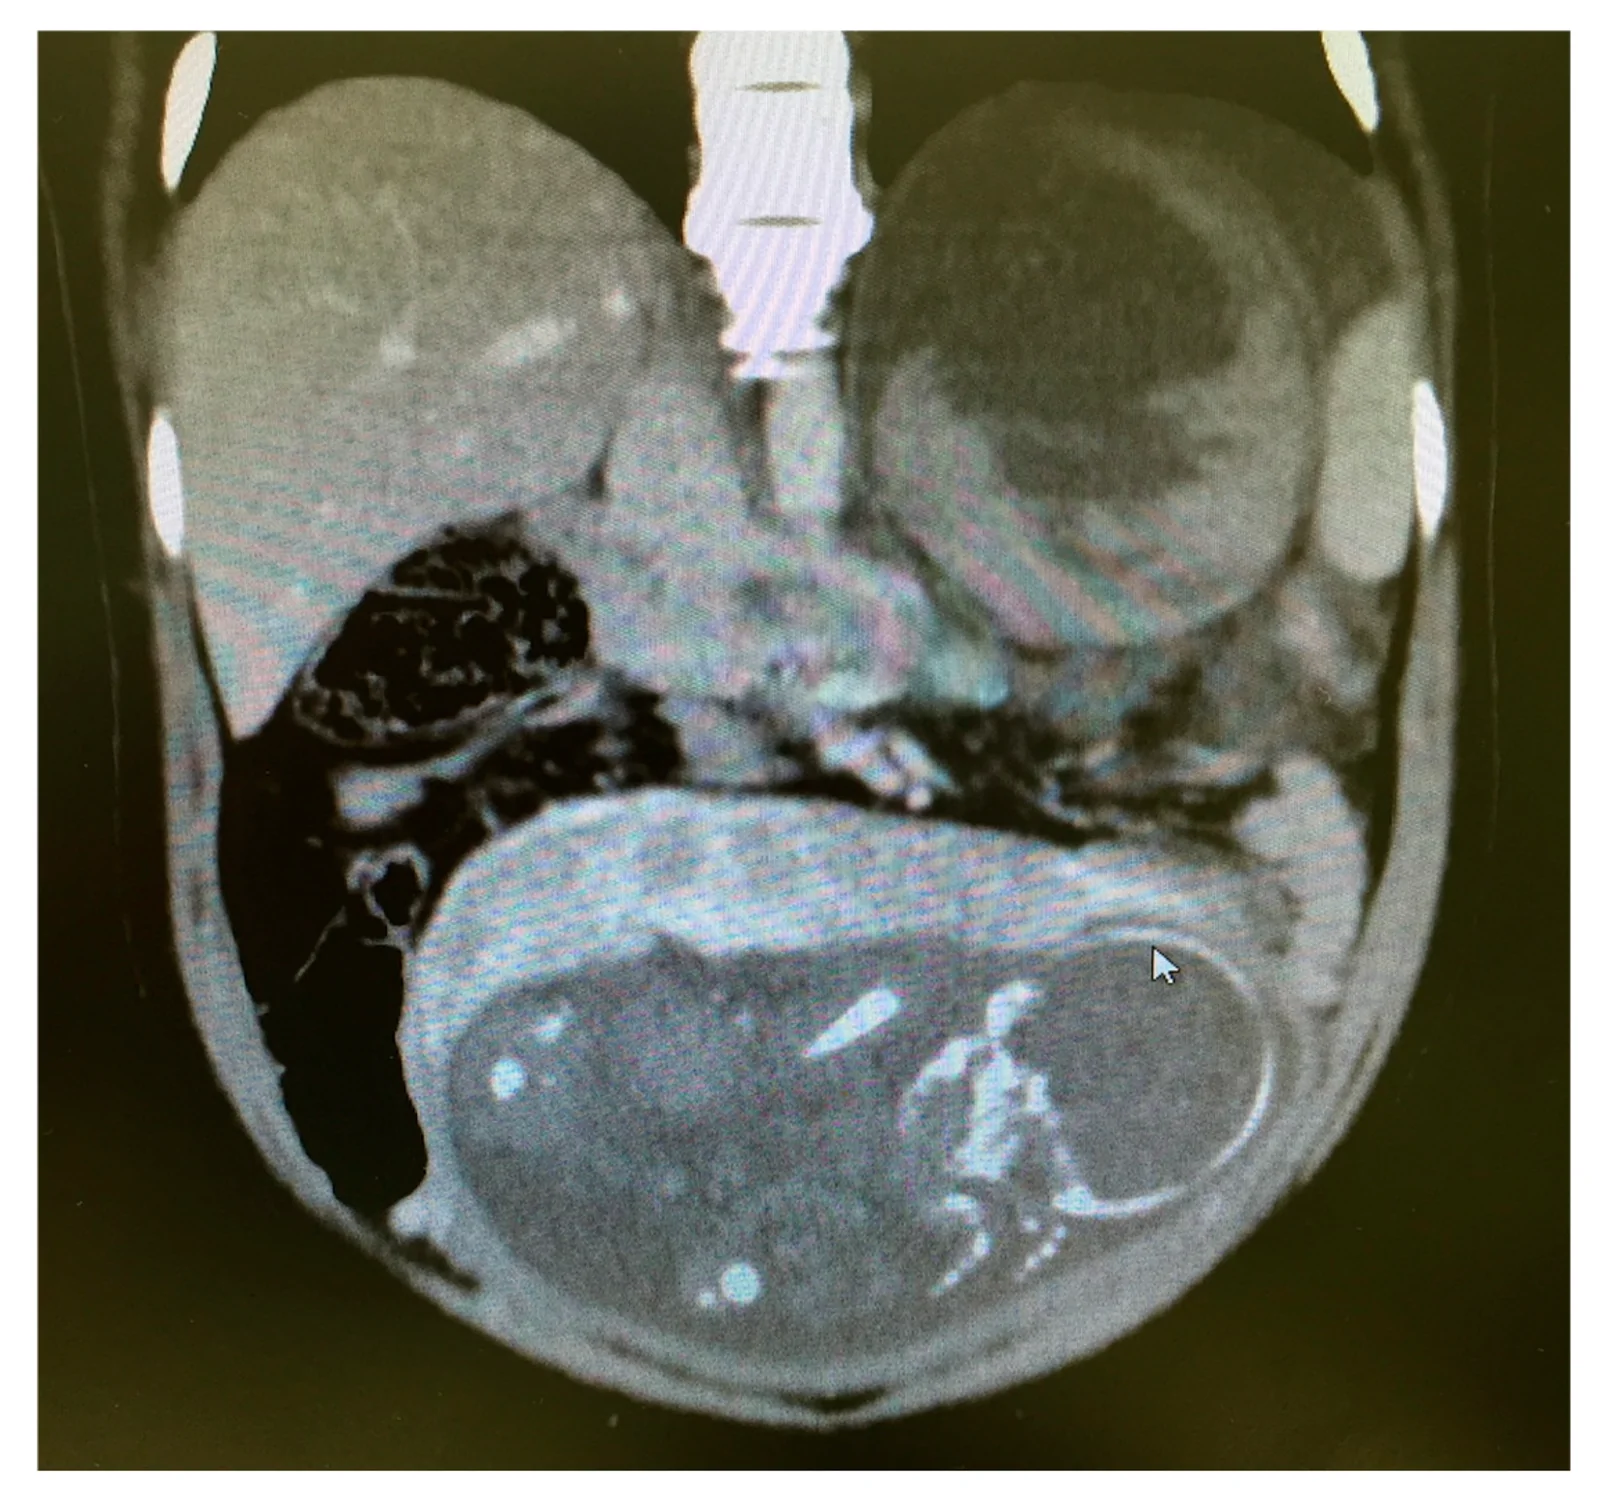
CT scan with IV contrast: A large well-circumscribed hematoma is seen in left suprarenal lesion of the left adrenal, most likely suggestive of left adrenal hemorrhage and a single intrauterine fetus (Case
